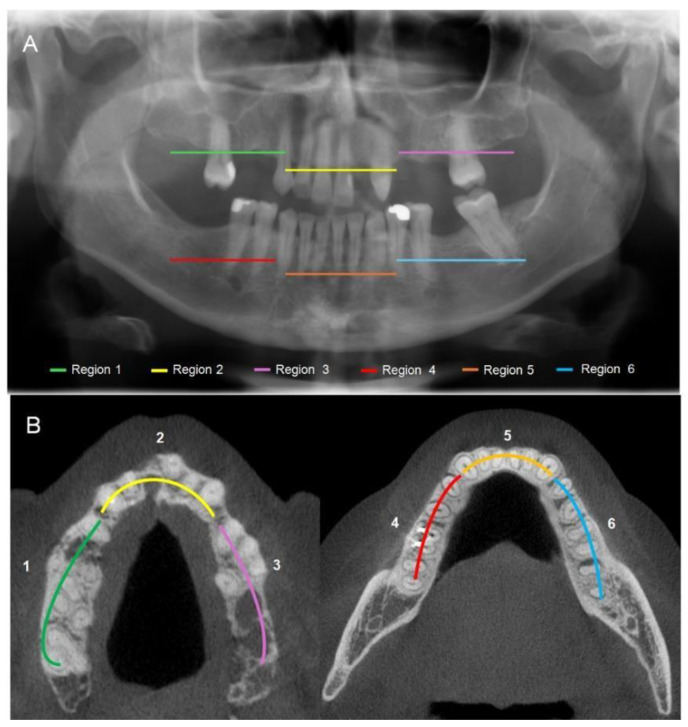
Analysis of panoramic radiography (A) and cone beam computerized tomography (B): the mandible and the maxilla were divided into six regions (maxilla: right, anterior, and left regions; mandible: right, anterior, and left regions).
